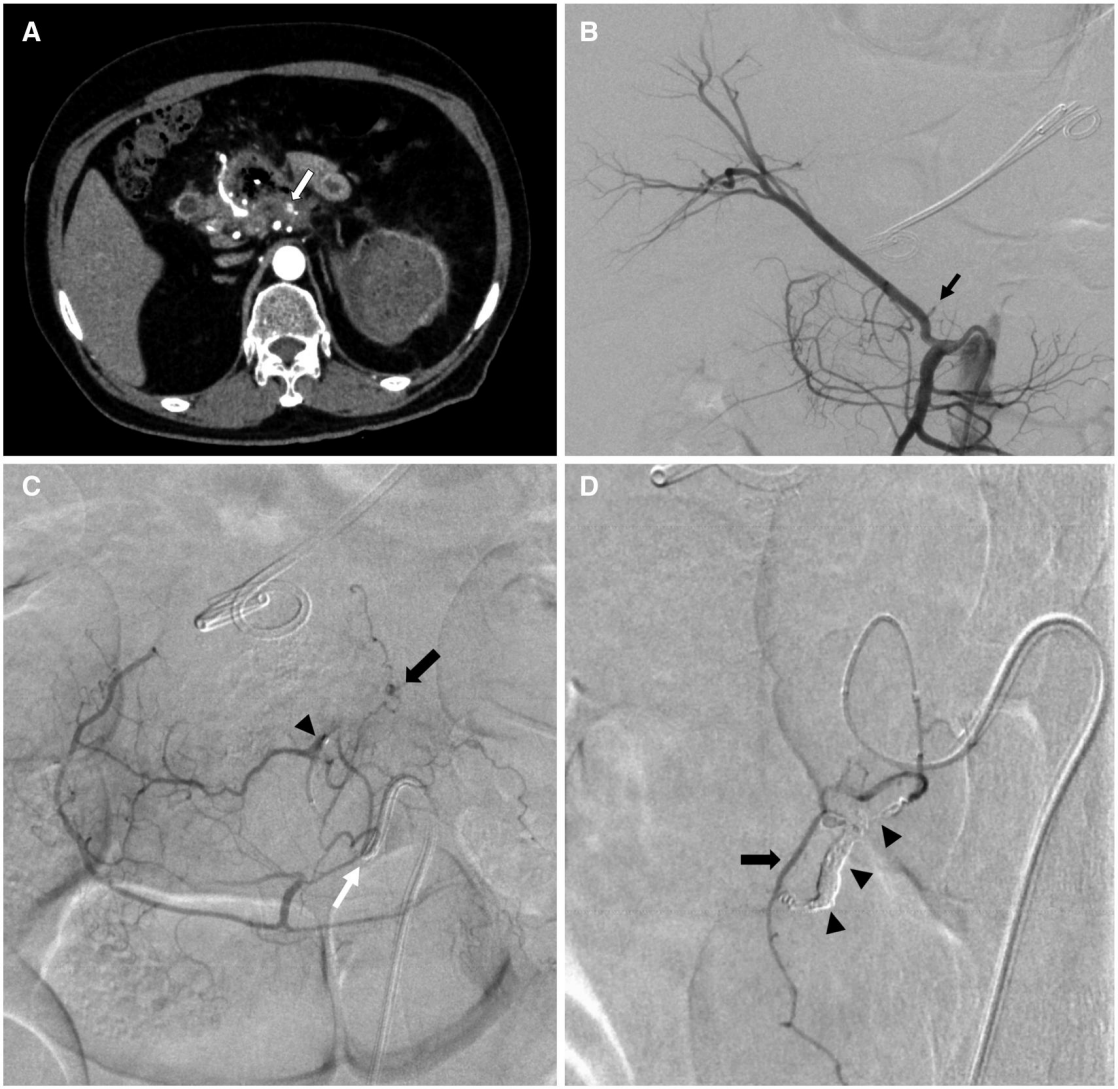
CT and angiographic images of the first transcatheter arterial embolization (TAE). (A) Contrast-enhanced CT showing a minute pseudoaneurysm around the abscess (arrow). (B) Angiogram of the superior mesenteric artery showing replaced right hepatic artery and the orifice of its first small branch (arrow). (C) Angiogram of the first branch of the replaced right hepatic artery shown in panel B (white arrow: orifice of the artery) demonstrating a small pseudoaneurysm (arrow) in the far distal portion of the left branch (arrowhead). (D) Angiogram of the distal part of the left branch indicated in panel C acquired using a 1.5-F microcatheter. A branch of the pancreaticoduodenal artery was occluded using microcoils to prevent off-target embolizations (arrowheads). The injection of the *N*-butyl-2-cyanoacrylate–lipiodol mixture resulted in minimal flow towards the small duodenal branch (arrow).
